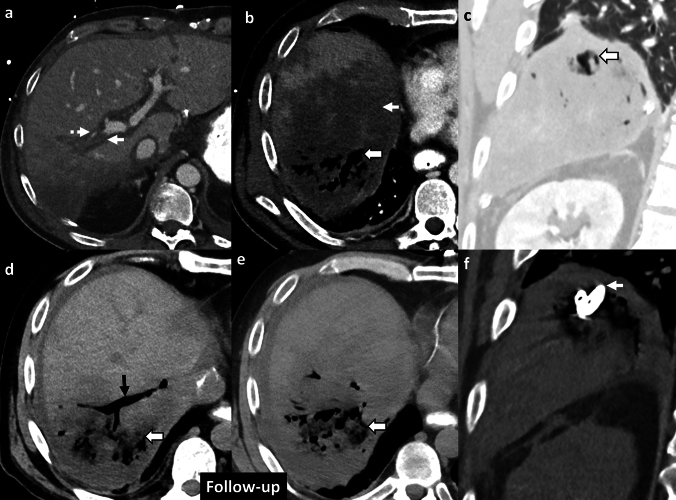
Emphysematous hepatitis or hepatic gas gangrene. A 43-year-old post-liver transplant patient with fever, sepsis, and abnormal liver function tests. Initial CT images a–c show thrombosed right portal vein (solid white arrow, a), diminutive right hepatic artery (dotted white arrow, a), hypoenhancing right hepatic lobe (solid white arrow, b) suggesting hepatic infarct with focal mottled air lucencies (block white arrow, b, c) within the infarcted liver consistent with hepatic gas gangrene. Note the distinct lack of fluid collection (block white arrow, c). Patient’s blood cultures were positive for *Enterobacter fecalis*, *Clostridium* species, and *Klebsiella* species. Follow-up CT images after one week d, e show increased extent of hepatic emphysema (block white arrows, d, e). Air also extends into the right hepatic vein (black arrow, d). Subsequently percutaneous drain was placed in focal emphysematous hepatitis (solid white arrow, f). The patient succumbed to sepsis despite aggressive measures, including redo surgery
